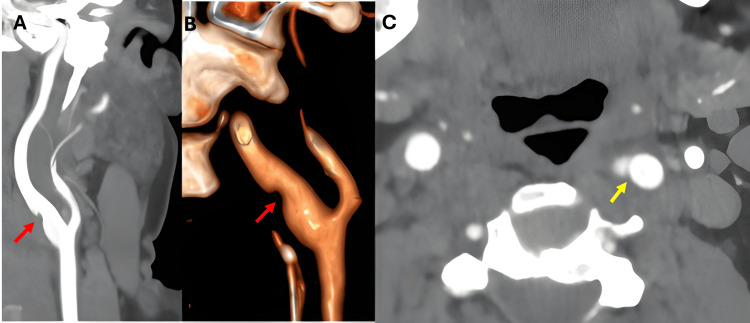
CTA of the neck showing the carotid web in the left ICA on coronal (A), 3D-reconstructed (B) views (red arrows), and axial view (C, yellow arrow). CTA: computed tomography angiography
